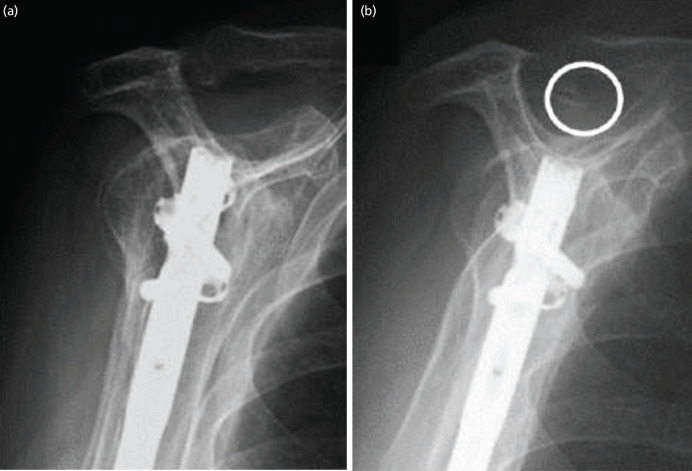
Plain radiographs of the right shoulder of a 79-year-old woman who underwent antegrade intramedullary nail fixation for humeral shaft fracture. (a) One-year after, and (b) five years after surgery. These radiographs show formation of the acromial spur at five years post-operatively.
